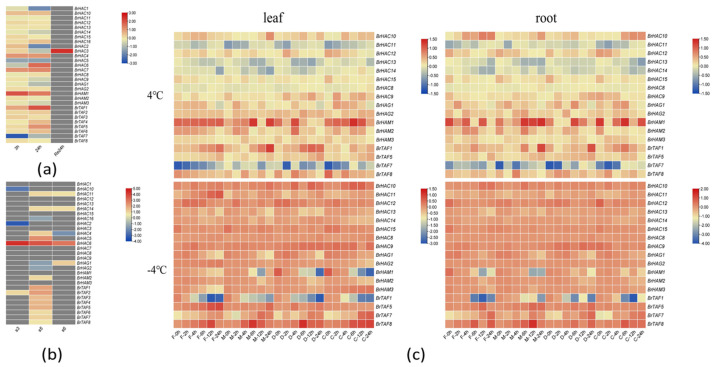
Expression analysis of *BrHATs* family members. (a). Transcriptome data of *BrHATs* family members in winter rapeseed (Longyou-7) at 4 °C. (b) Transcriptome data of *BrHATs* family members in winter rapeseed (Longyou-7) at different overwintering stages; sampling date: S3 (24 November 2019), S5 (4 January 2020), and S6 (25 April 2020). (c). Expression analysis of *BrHATs* family members in leaves and roots under low-temperature stress. The gray rectangles in Figure 4a,b indicate that no significant differential expression of this gene occurred under this treatment compared to the control. “C” is “CT-2360,” “M” is “MXW-1,” “D” is” DT-9,” and “F” is “2018-FJT.”.
